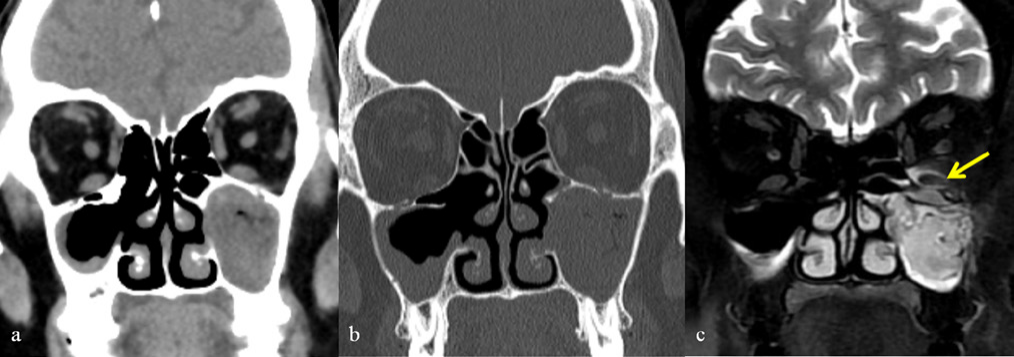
Coronal CT (soft tissue and bone window) and MRI image of mucormycosis. (a) and (b) showing complete opacification of left maxillary sinus. No orbital fat stranding detected. Coronal T2FS MR image (c) showing inflammatory changes around inferior rectus (yellow arrow) indicating orbital cellulitis.
